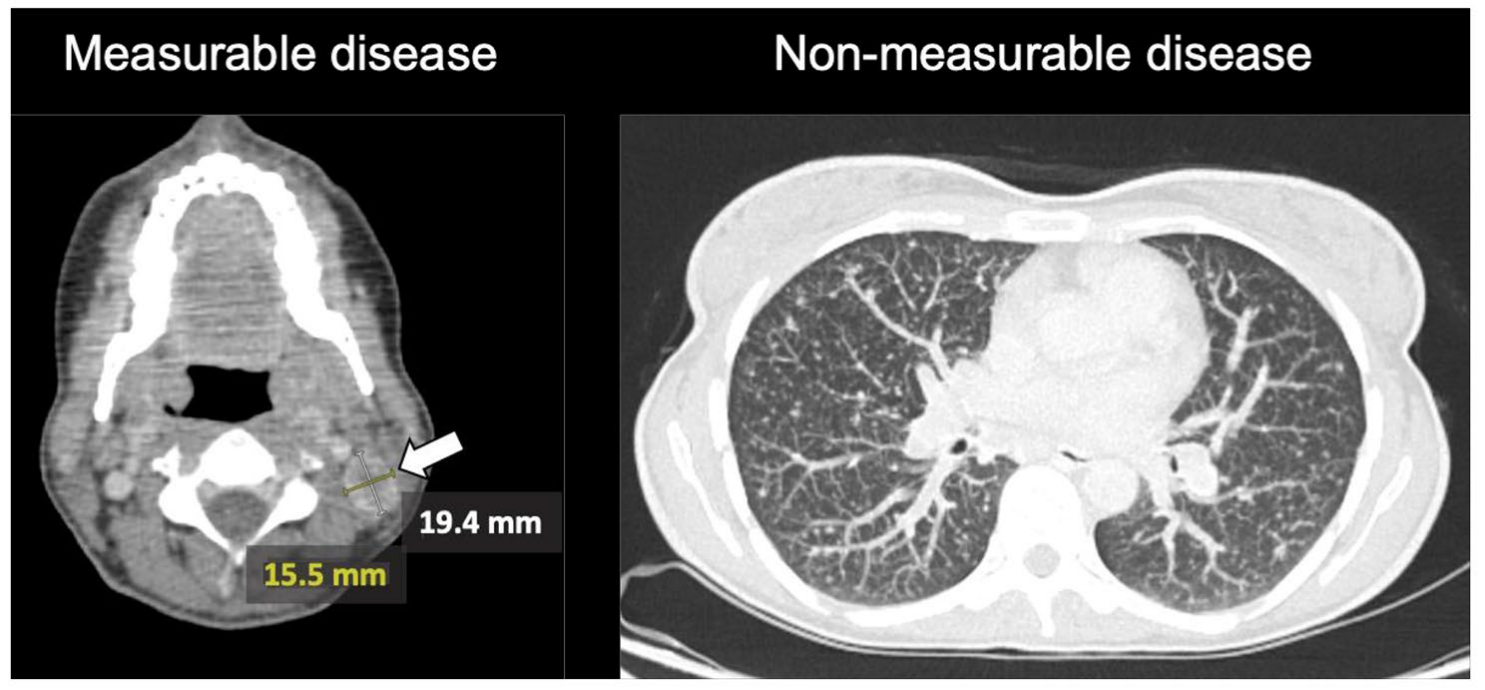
Example of MD non-representative of the overall tumor burden in contrast enhanced CT A female patient with resected thyroid cancer and a solitary measurable cervical lymph node metastasis on the left side (Level IIb) measuring 15,5 mm in the short axis (left image, white arrow). The CT-scan of the thorax in lung window (right image) of the same patient shows presence of extensive small nodular pulmonary metastases classified as non-measurable due to small tumor size of less than 10 mm
